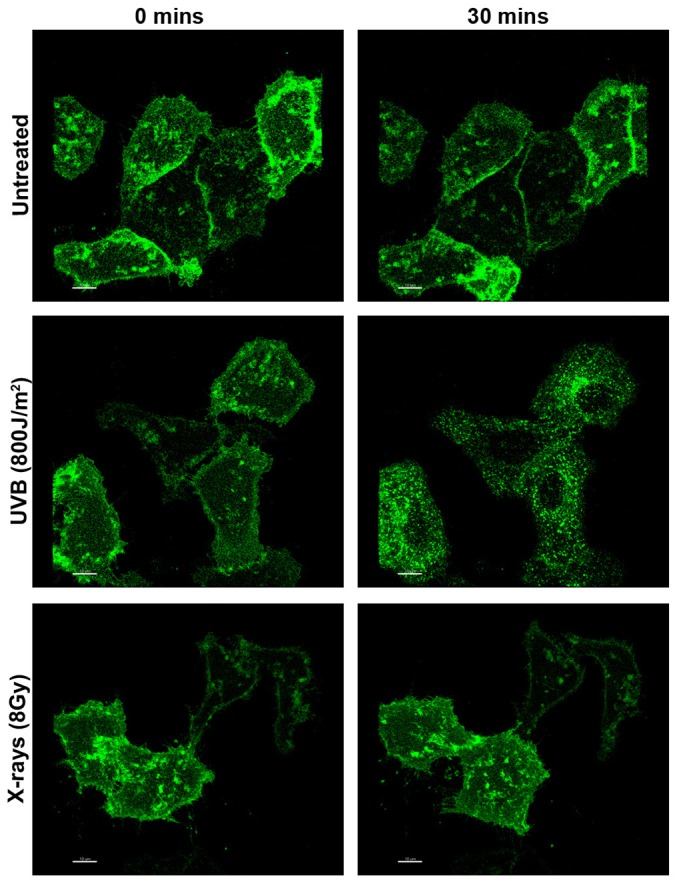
UVB, but not X-rays, induce endocytosis of EGFR–GFP in HeLa cells. HeLa cells transiently transfected with EGFR–GFP were left untreated, or treated with UVB (800 J/m^2^) or X-rays (8 Gy). Cells were imaged for 30 min with the first frame taken at 3.5 min and 7.5 min after UVB and X-ray irradiation respectively, and images were taken every 90 s (see Movies 1–3). In untreated and X-ray-treated cells there is little change in EGFR–GFP distribution but EGFR–GFP redistributes from the cell surface to the cell interior after UVB treatment. Scale bars: 10 µm.
